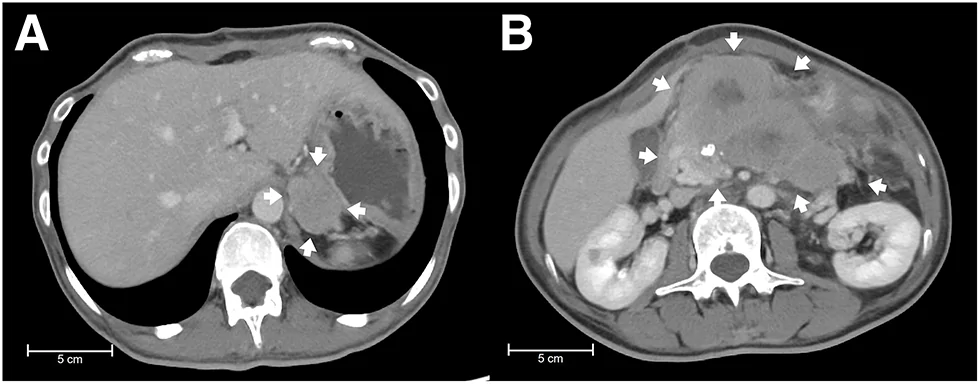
Postoperative imaging. (A, B) CT revealed peritoneal recurrence 3 months after surgery (arrows).
